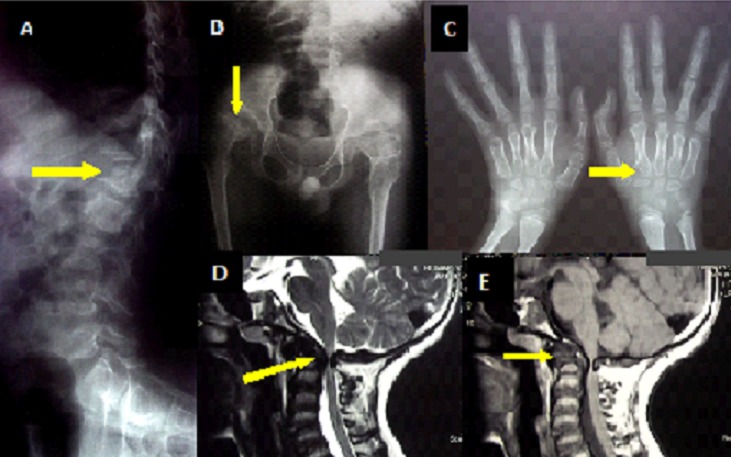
X-ray appendicular radiographs showing a platyspondyly (image A), acetabular dysplasia (image B) and metacarpal irregular proximal ends (image C). Images D and E, showing the marked atlanto-axial instability, and spinal cord compression
